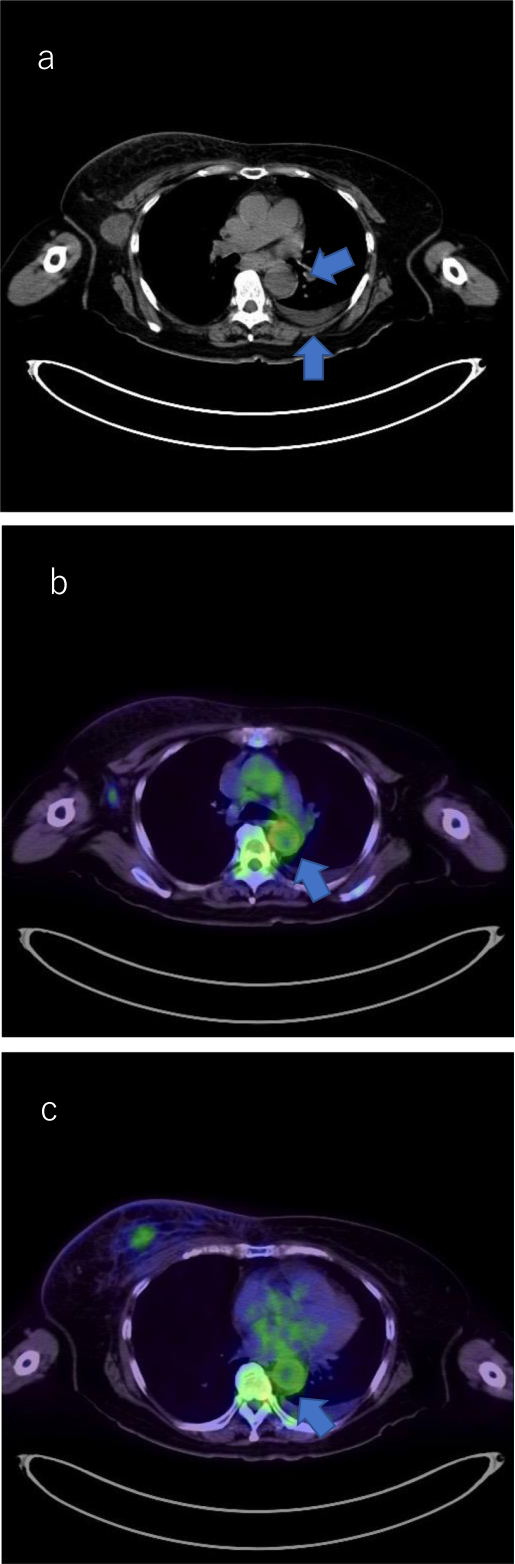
FDG-PET/CT shows the thickened wall of the thoracic aorta and left pleural effusion (a) with abnormal uptake of FDG (b, c)
